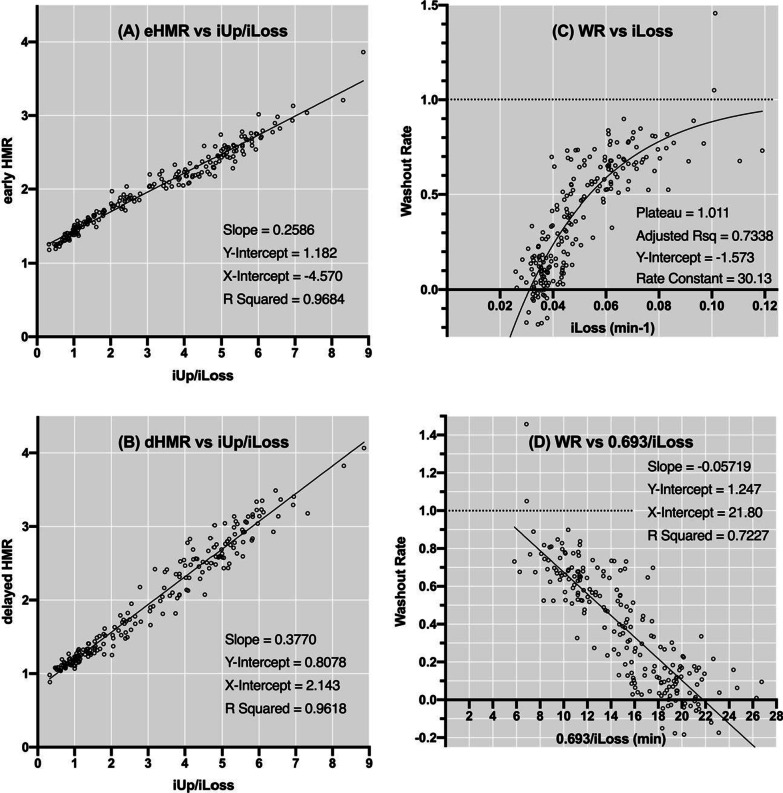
The results of regressions between the existing indices and new indices of ^123^I-MIBG myocardial scintigraphy. Linear regressions between iUp/iLoss and the early (eHMR) (A) and delayed HMR (dHMR) (B) are shown. A nonlinear regression with an exponential monomolecular growth model between iLoss and WR (C) can be converted to a linear regression between 0.693/iLoss and WR (D)
